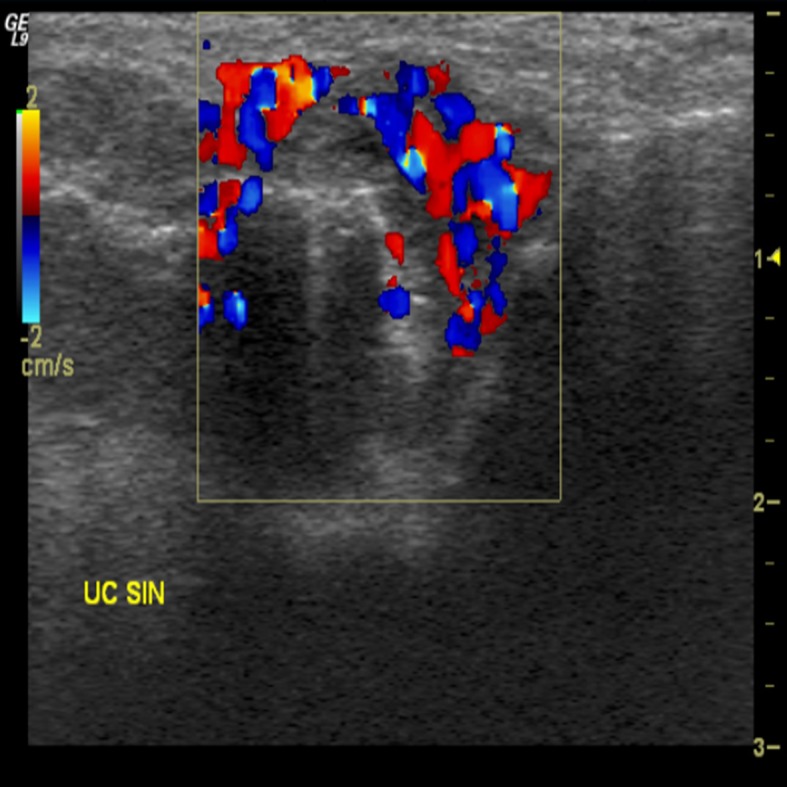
Vascularization in ulnocarpal joint – group 3: more Doppler signals in vessels on the surface larger than the half of the synovial size.
